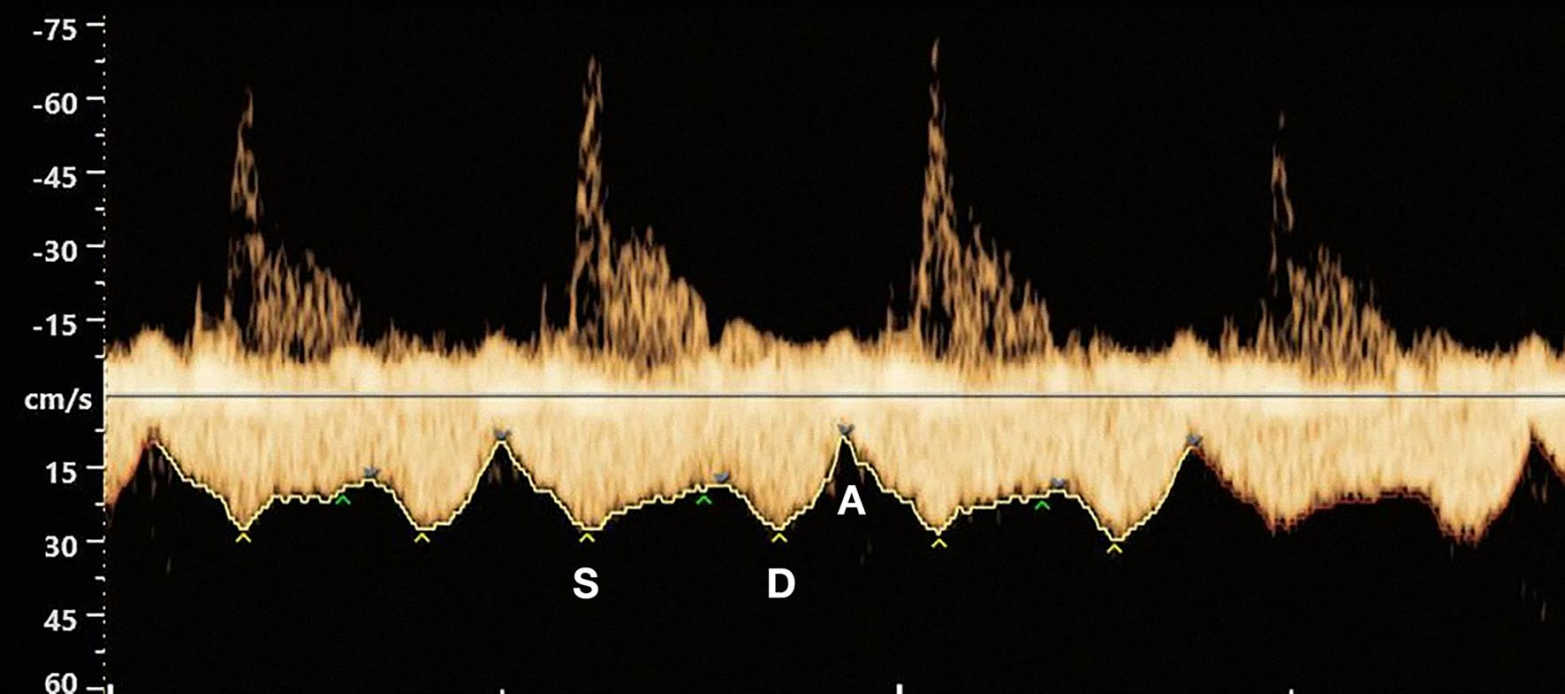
Fetal pulmonary veins’ waveform: the normal waveform of the fetal pulmonary veins is characterized by a pulsatile flow: S-wave, during ventricular systole (S); D-wave, during early ventricular diastole (D); A-wave, a nadir at the end of diastole (A); the simultaneous recording of Doppler waveforms from pulmonary artery allows the qualitative assessment of the relationship of atrial to ventricular contraction
